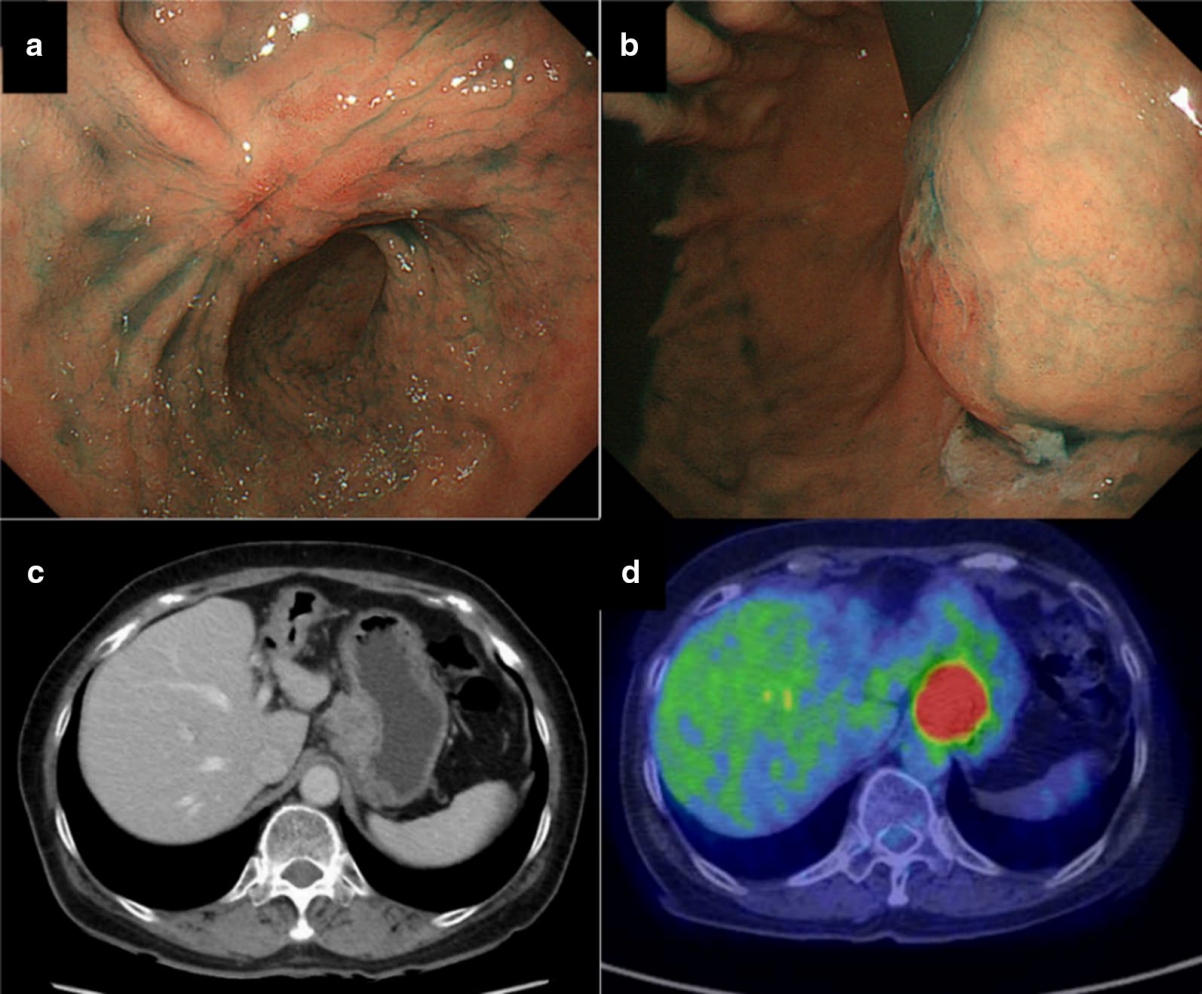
Preoperative findings. a, b EGD found ESD scar at the lesser curvature of antrum and a SMT at the lesser curvature of cardia. c CT shows an approximately 30-mm, high density, round mass at the lesser curvature of upper gastric body. d Uptake of fluorodeoxyglucose seen on PET–CT
